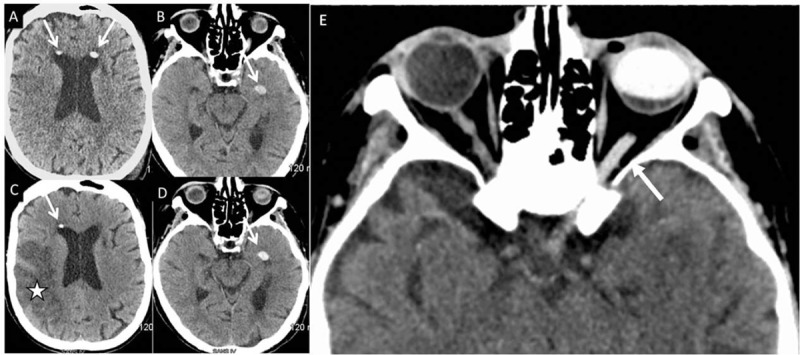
Day 0 (A, B) and day 2 (C, D) axial noncontrast-enhanced brain CT scans (same sections: A = C; B = D) showing moving intraventricular hyperdensities (arrows) and a cerebral infarction (star). E: hyperdensity of the left optic nerve (arrow) and the left ocular globe. CT = computed tomography.
